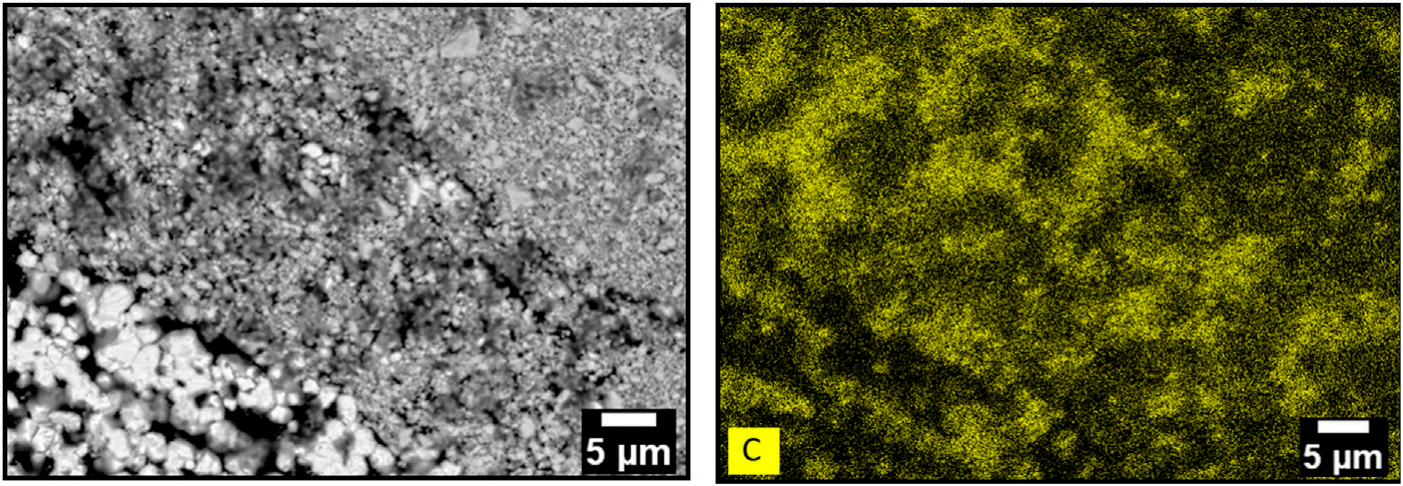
X-Ray elemental mapping analysis of a cross-section of a sorbent bead calcined at 500°C.
